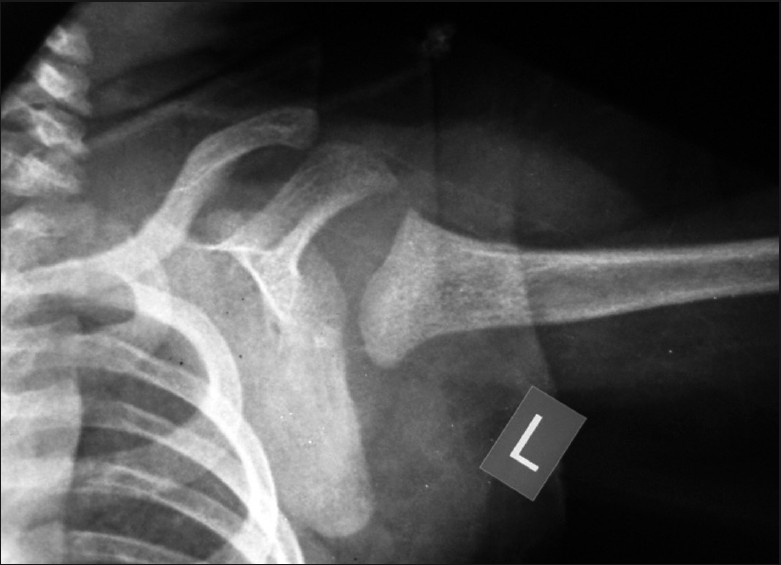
X-ray picture of the left shoulder showing an anteroinferior dislocation
